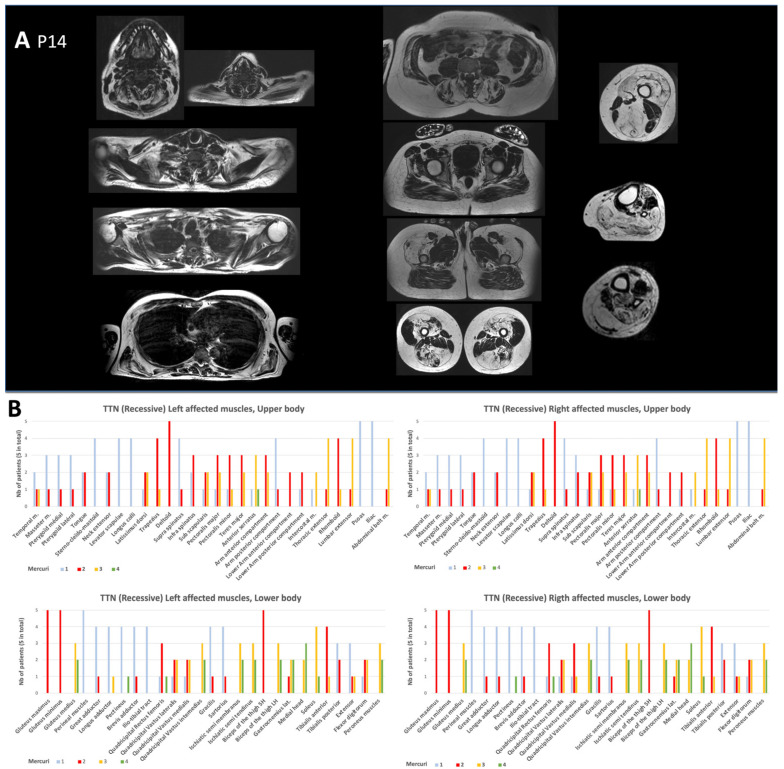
MRI data. (A) Patient P14 MRI analysis, selection of axial T1 weighted sections of a patient with a recessive form of titinopathy. Muscle damage with fat infiltration is disseminated; the most preserved muscles are the supraspinatus, psoas–iliacus, gluteus maximus, great and long adductors, and short head of the biceps femoris. Fat content in muscles is more pronounced in anterior and posterior thighs and legs. (B) Assessment of muscular damage to all four limbs using the Mercuri scale. Fatty replacement varies widely from muscle to muscle and from patient to patient. However, involvement predominates in proximal muscles: in the upper limbs, the deltoids, trapezius, rotator, and scapula fixator muscles are the most affected, whereas in the lower limbs, involvement predominates in the glutei muscles, in the thighs and legs, although the sartorius and gracilis muscles are spared.
